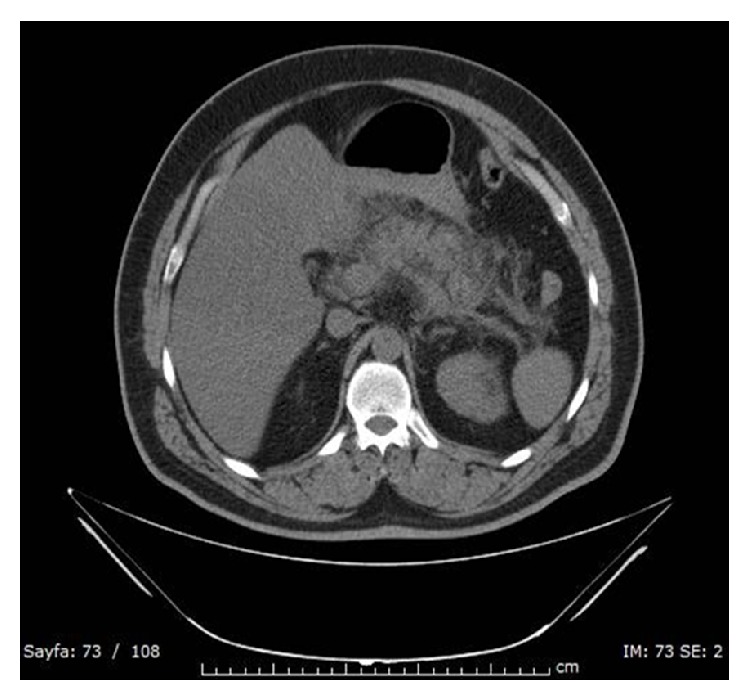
Computed tomography of abdomen showed edematous characteristics in peripancreatic region.
